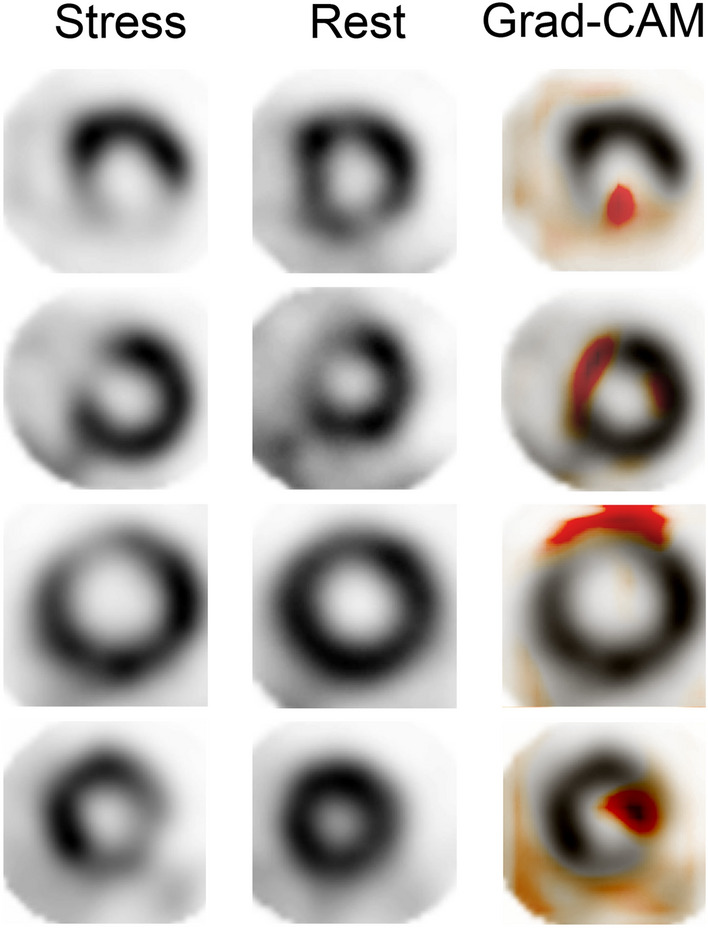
Visualization of diseased area using Grad-CAM. Representative Grad-CAM images showing lesions in MPI SPECT images of coronary artery disease. Short axis image in the stress condition (left), rest condition (center), and Grad-CAM merged image in the stress condition (right). MPI SPECT, single-photon emission computed tomography for myocardial perfusion imaging.
